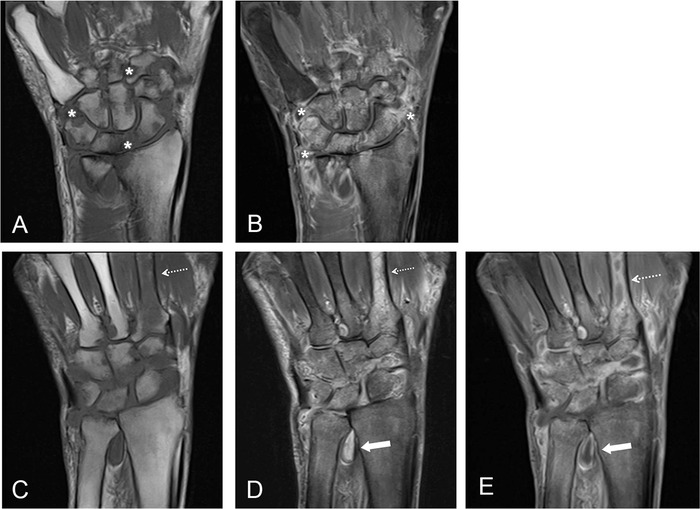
Most recent magnetic resonance imaging examination at the carpal level with (A) coronal T1 and (B) coronal postcontrast T1 fat saturation sequences with inclusion of the metacarpals on (C) coronal T1, (D) coronal short T1 inversion recovery (STIR), and (E) coronal post-contrast T1 fat saturation sequences. Views A and B show joint space narrowing, osseous erosions (asterisks), and synovial enhancement (asterisks) throughout the carpals. Dashed white arrows show marrow edema (views C and D) and enhancement (view E) of the second metacarpal concerning for osseous involvement of the adjacent infectious process within the carpals. Additionally, complex enhancing fluid is seen at the distal radioulnar joint (solid arrows in views D and E).
